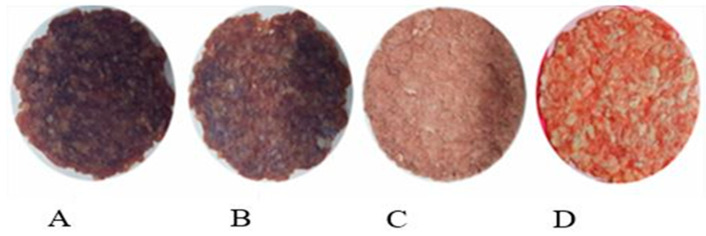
Appearance of meat samples subjected to different drying techniques: (A) vacuum drying (VD) at 45 °C, (B) ultrasonic vacuum drying (USV) at 45 °C, (C) freeze-drying (FD), and (D) fresh sample. The superior color preservation in the FD sample is evident. Adapted from [135]. under the terms of the CC BY license. Copyright 2019, Aksoy, A.; Karasu, S.; Akcicek, A.; Kayacan, S.
